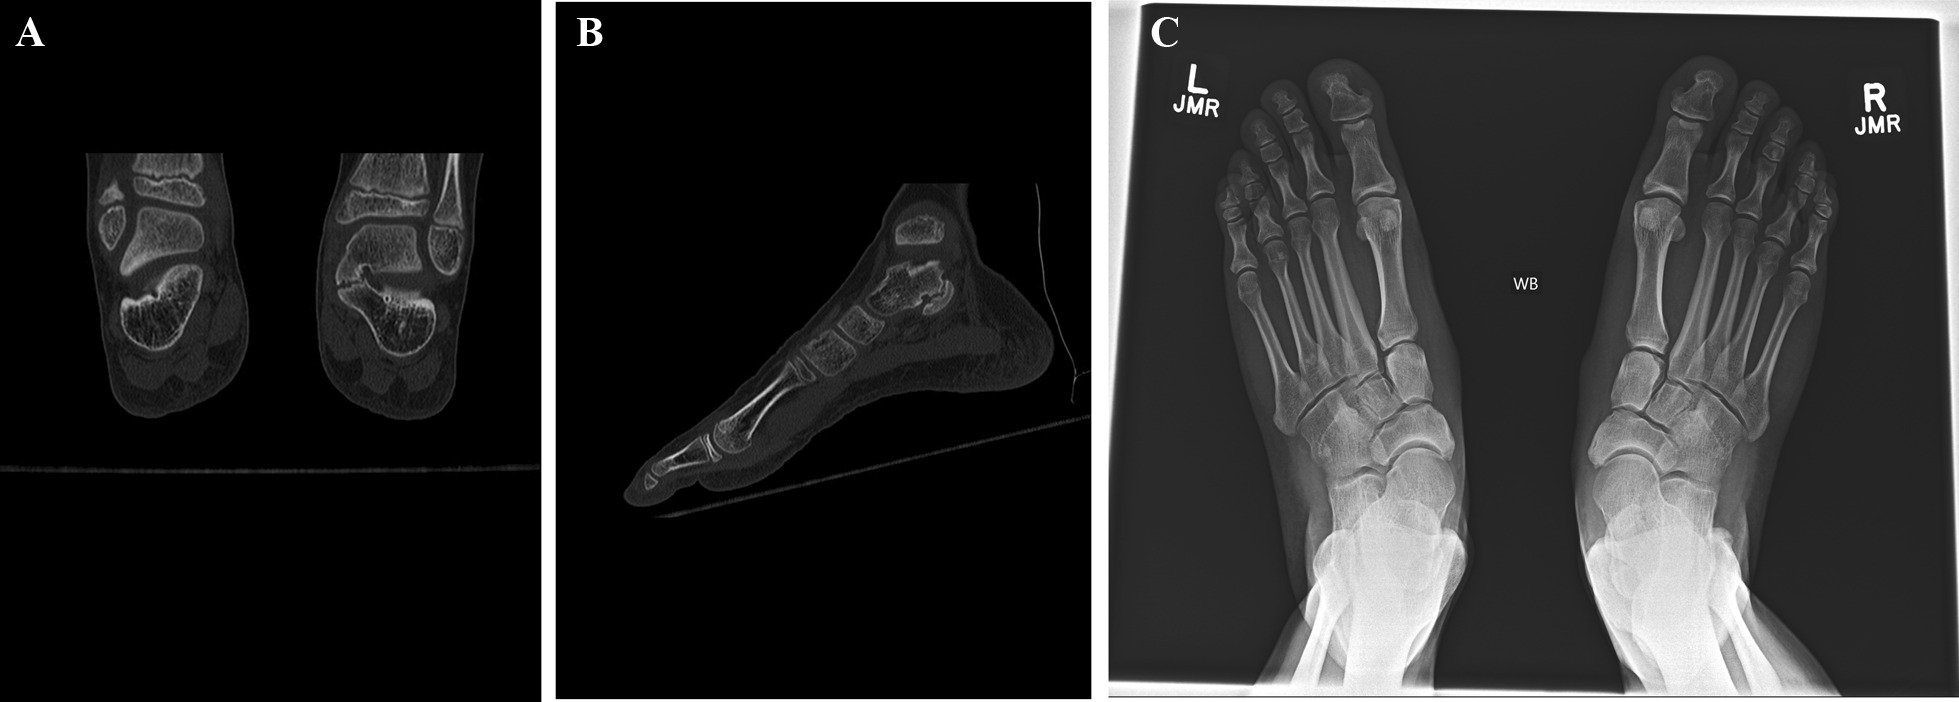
A Preoperative radiograph with CN coalition. B Preoperative medial oblique plain radiograph with CN coalition. C Preoperative lateral plain radiograph with CN coalition
